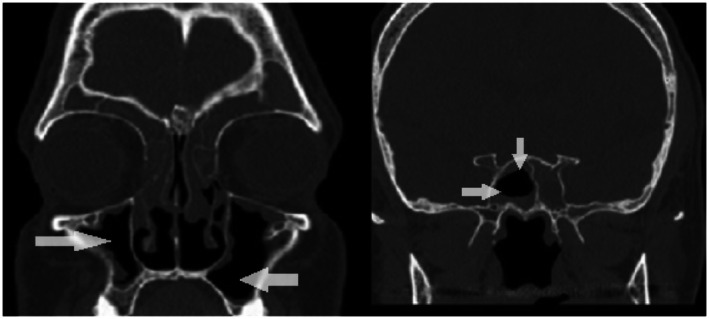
Coronal paranasal sinus CT scan, soft‐tissue window, Day 17. The image demonstrates a conspicuous reduction in maxillary sinus opacification and partial sphenoid improvement. White arrows and labels indicate areas of signal clearance corresponding to early radiologic response (Lund−Mackay score improved from 18 to 14).
